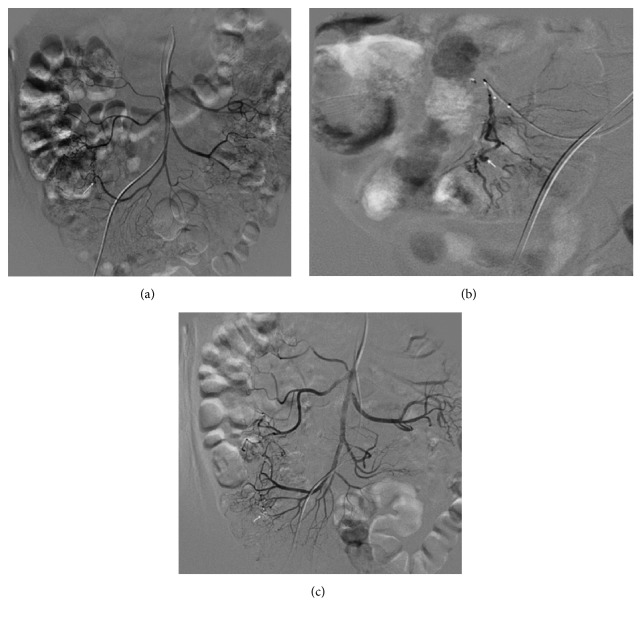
The radiography in the patient (Case 5) with lower gastrointestinal bleeding and angiodysplasia of ascending colon. (a) The preoperative radiography shows proximal vascular malformation of ascending colon (as showed by the arrow). (b) The superselective radiography showed the enlarged tortuous vessel and microaneurysm (as showed by the arrow). (c) The postoperative radiography showed that the abnormal sign disappeared (as showed by the arrow).
